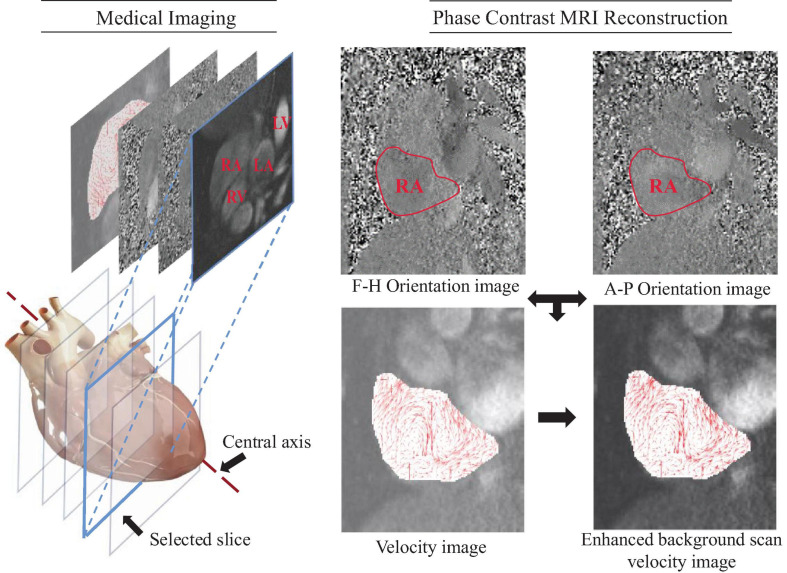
A representation of the MRI scan images through the heart, and PC-MRI based velocity images in a cardiac chamber. The PC-MRI pertains to the Foot-Head (F-H) and the Anterior-Posterior (A-P) orientations, respectively, the intensity of the whose pixels indicates the magnitude of the velocity component in the specified orientation. Combining two orthogonal velocity-encoded images can yield a velocity flow field.
